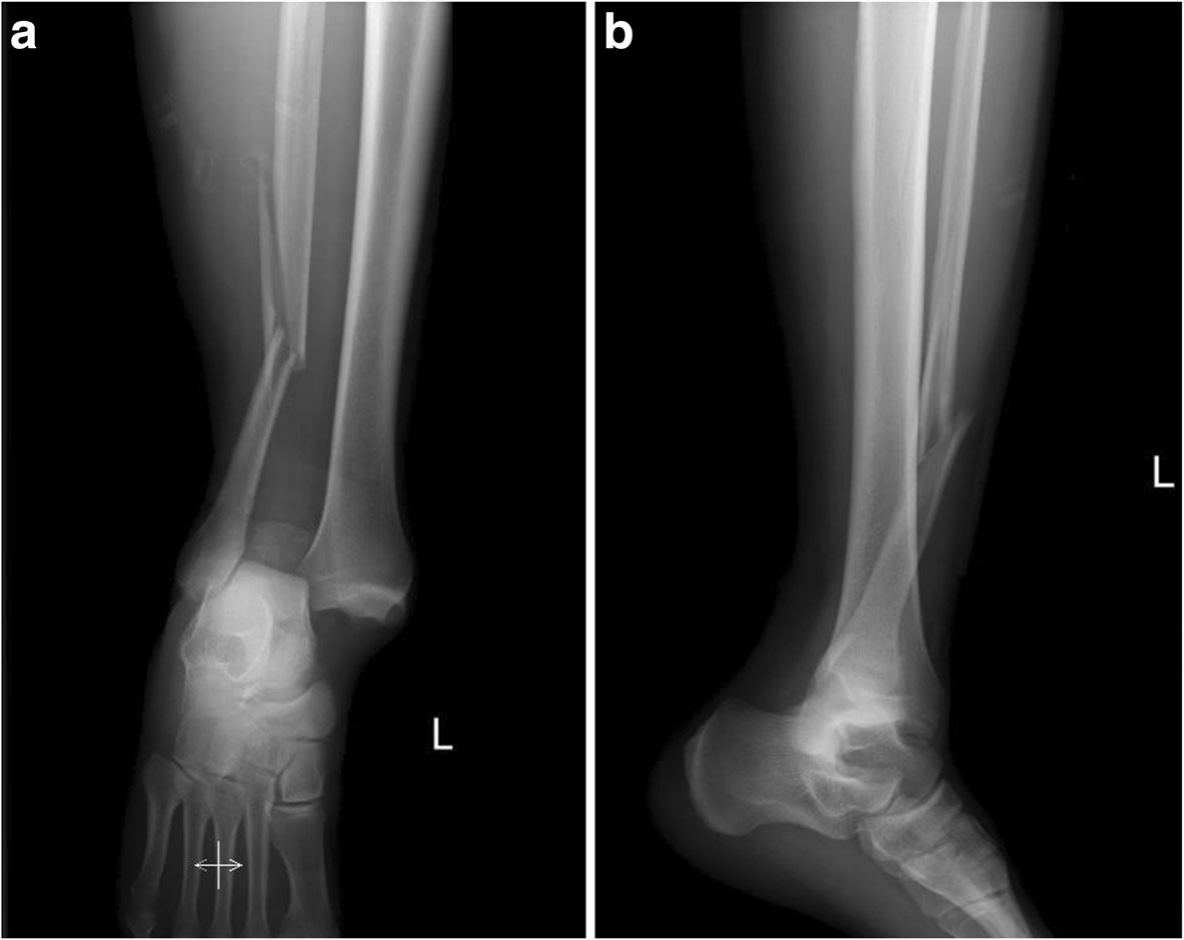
The anteroposterior (a) and lateral (b) radiographs of typical Logsplitter injury
